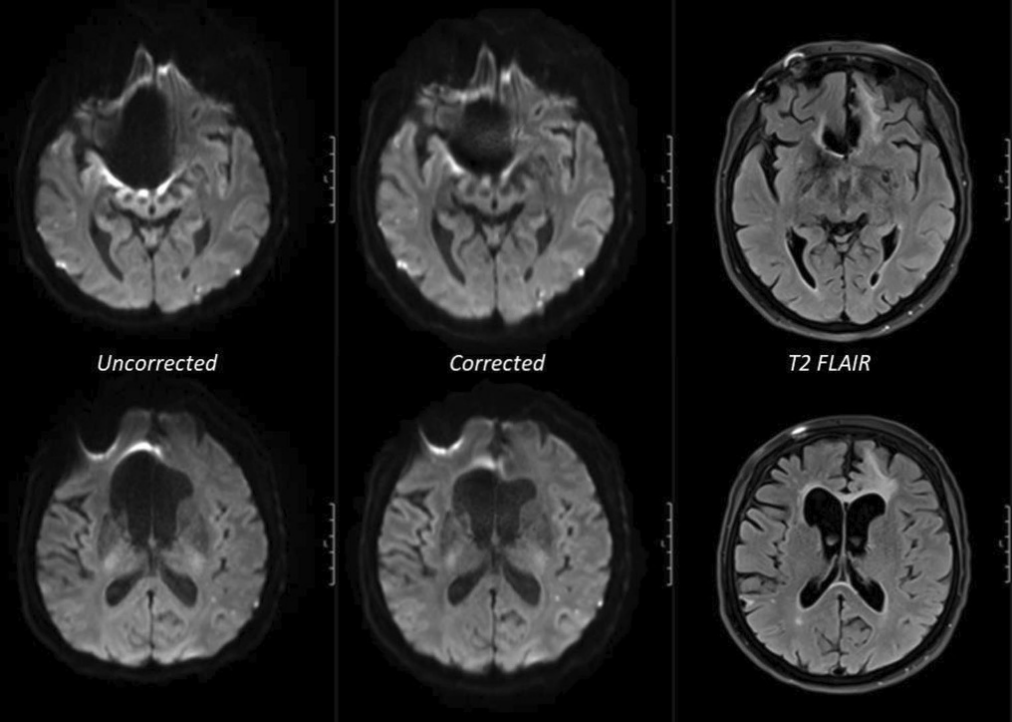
Examples of axial MRI scans of two different slice positions in the same patient with a surgically clipped aneurysm of the right anterior cerebral artery (*upper row*): substantially reduced artifact around the metal clip in corrected EPI diffusion. (*lower row*): consequentially improved geometry of the right anterior horn of the lateral ventricle due to the reduced metal-induced artefact. Matched T2 FLAIR images are provided for comparison
